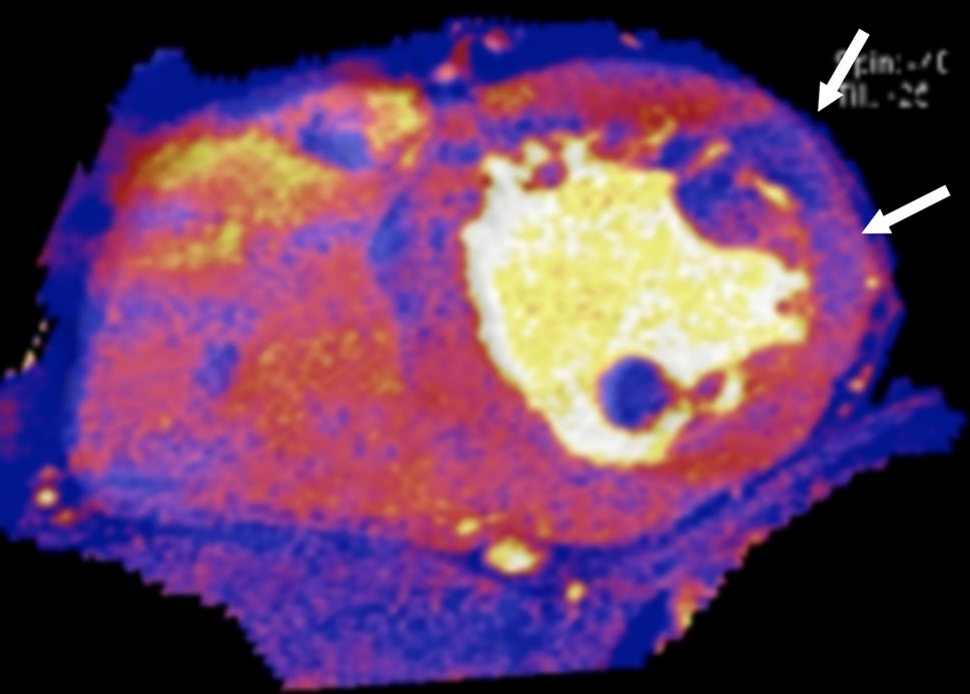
A dual-energy CT scan overlaid with iodine in the short-axis plane obtained from a static stress myocardial CTP scan showing a perfusion defect in the mid anterolateral septum (arrow). *CT* computed tomography, *CTP* computed tomography perfusion
